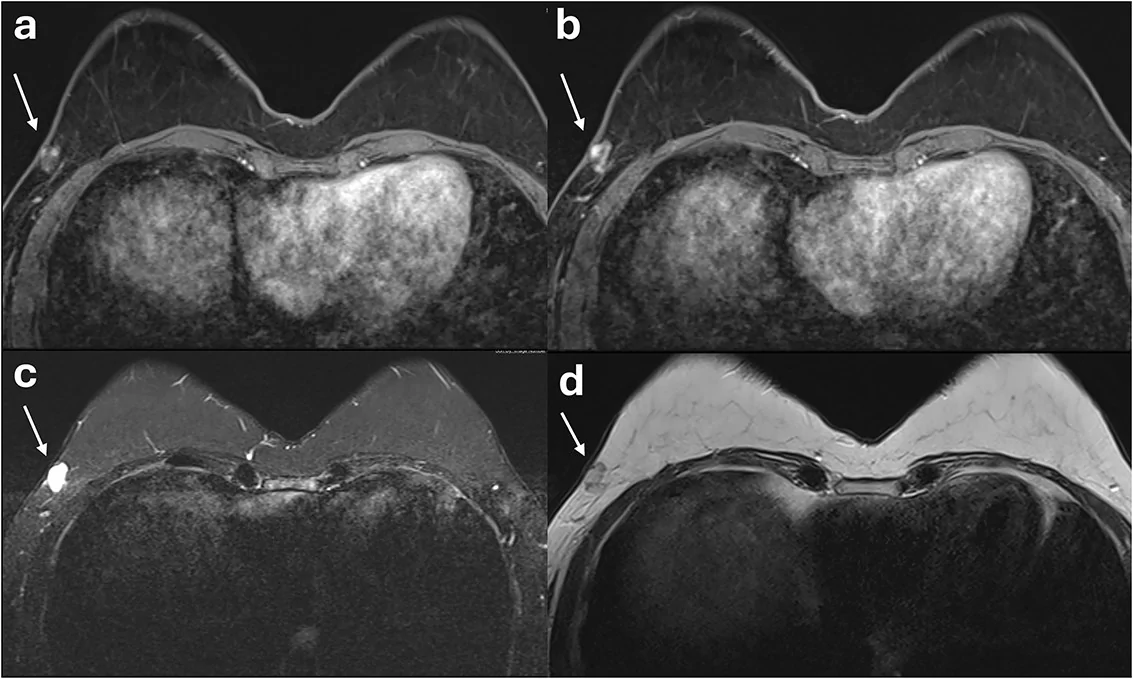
(a) T1-weighted MRI in the early phase revealed a 15 × 8 mm lesion in the lower lateral quadrant of the right breast (arrow), (b) T1-weighted MRI in the late phase with a gradual contrast accumulation (arrow), (c) STIR MRI sequence showing a very high signal intensity of the mass (arrow), (d) T2-weighted MRI sequence showing an intermediate signal intensity throughout the lesion in the lower lateral part of the right breast (arrow).
